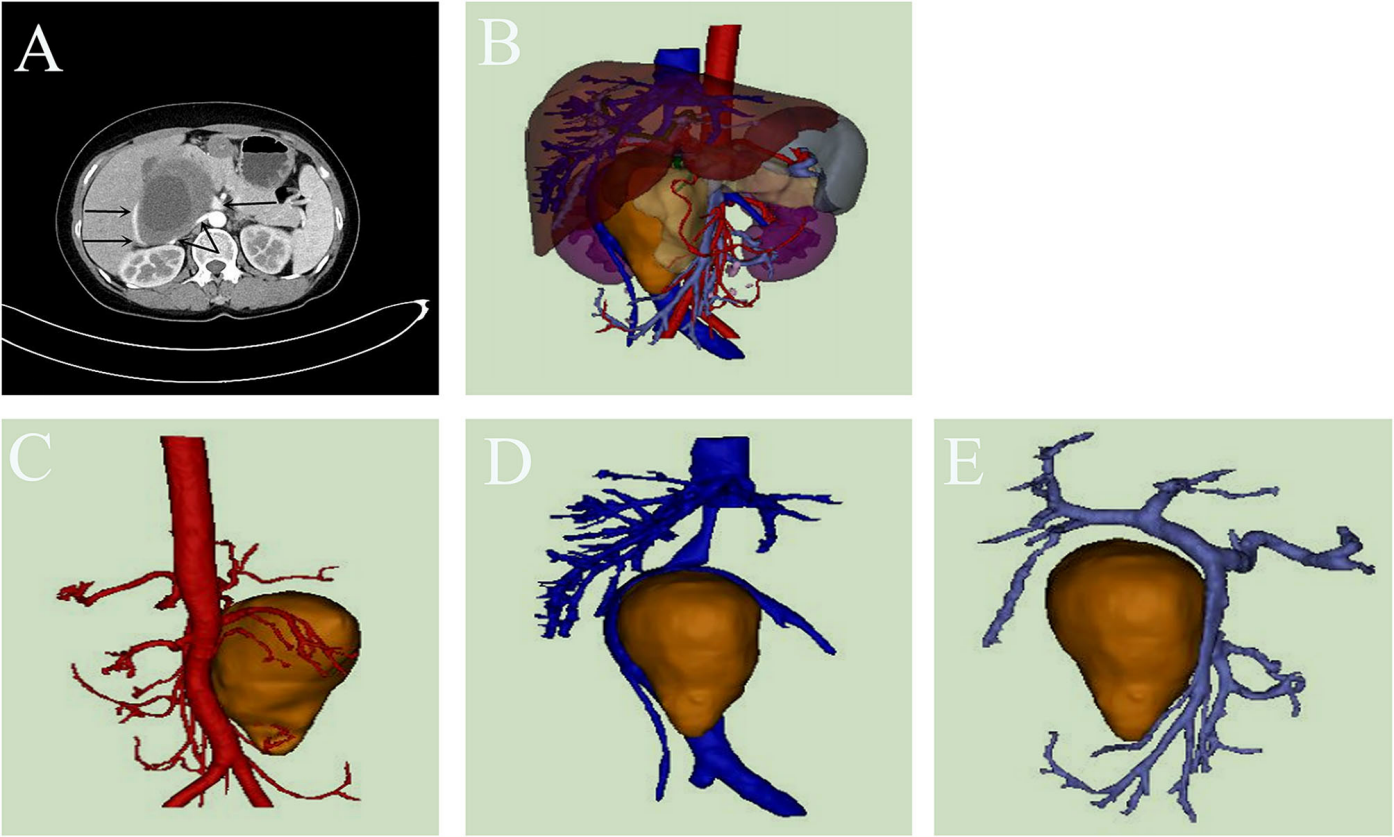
(A–E) The enhanced Computed Tomography (CT) and three-dimensional reconstruction performance of Case 2.
